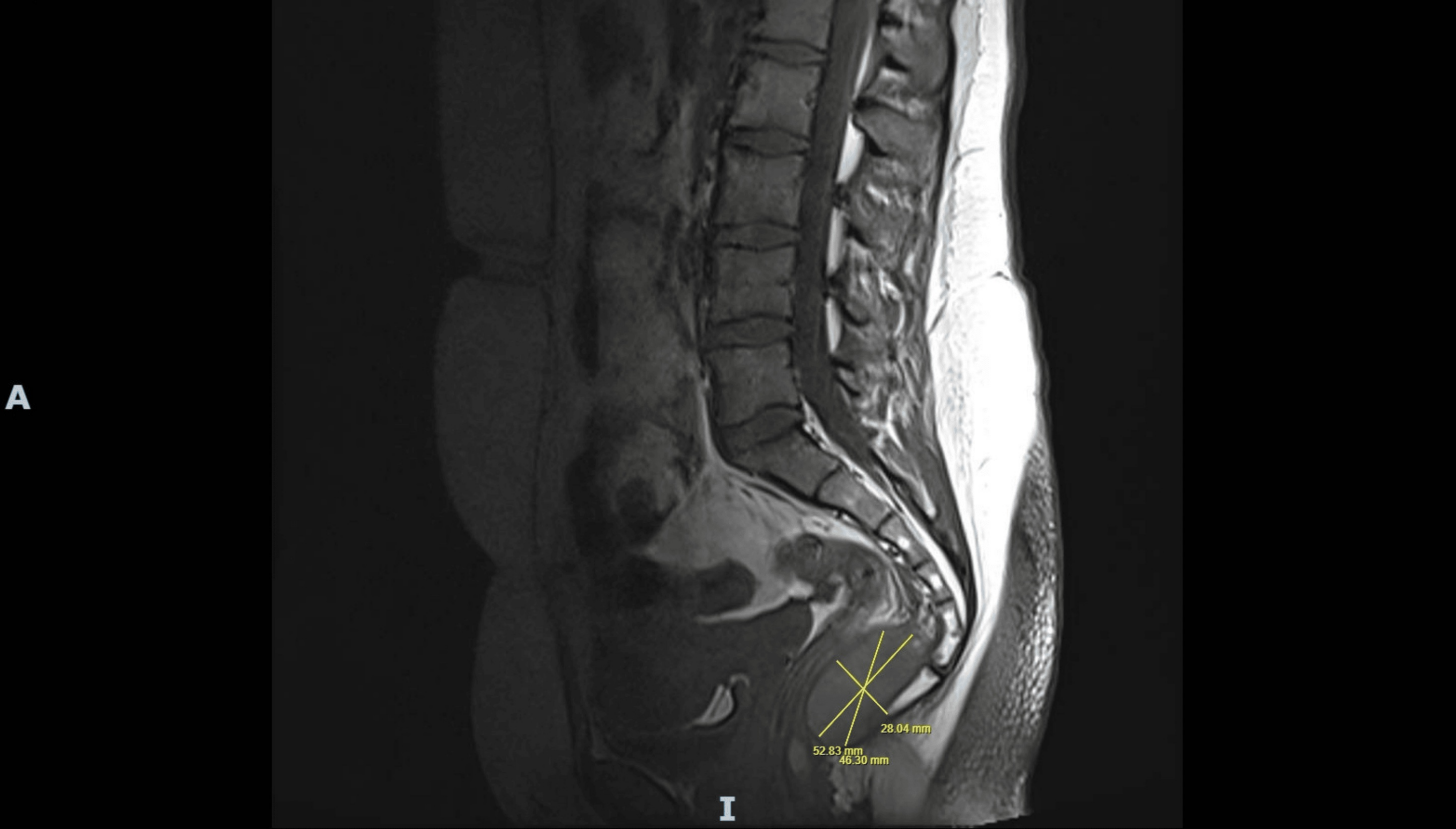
Sagittal MRI of pelvis (2025) demonstrating presacral cystic lesion with thickened walls and internal septations
